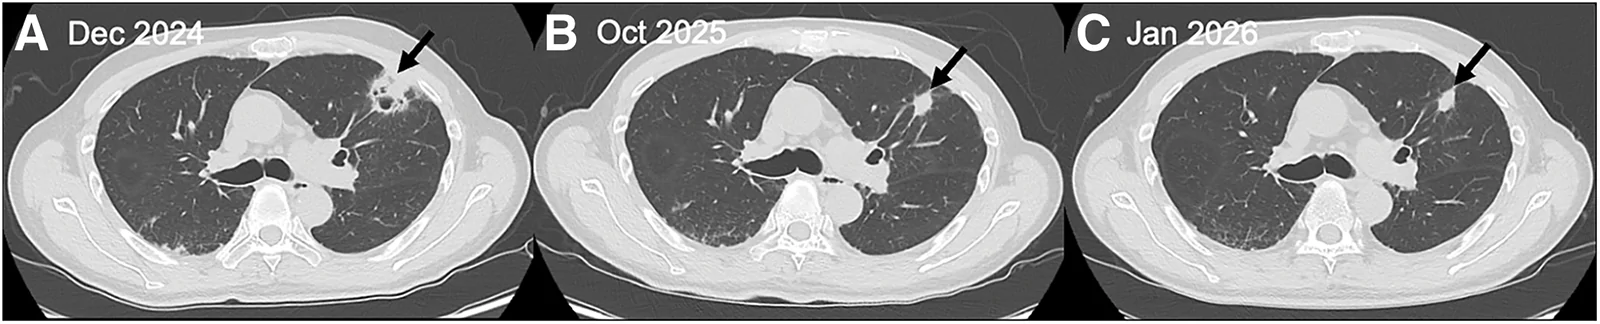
Serial Chest CT. Chest CT images obtained at baseline (A; December 2024), during pembrolizumab therapy (B; October 2025), and before the onset of gastrointestinal bleeding (C; January 2026). The arrows indicate the left S3 lung lesion, which showed reduction followed by sustained control.
